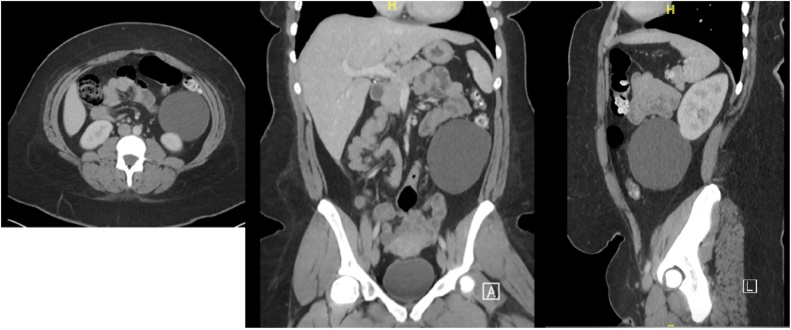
CT scan of mass measuring 8.0 × 8.4 x 9.4 cm: axial, coronal, and sagittal views.
